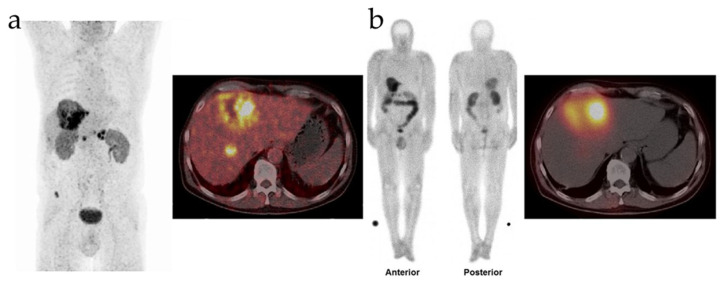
Therapeutic comparison of ^177^Lu-labeled targets. (a) PET images and a transverse PET/CT image captured with ^68^Ga-FAP-2286 before treatment for the patients. (b) Anterior and posterior views and transverse SPECT/CT images at 48 h after ^177^Lu-FAP-2286 injection. Figures from Baum et al. [221].
